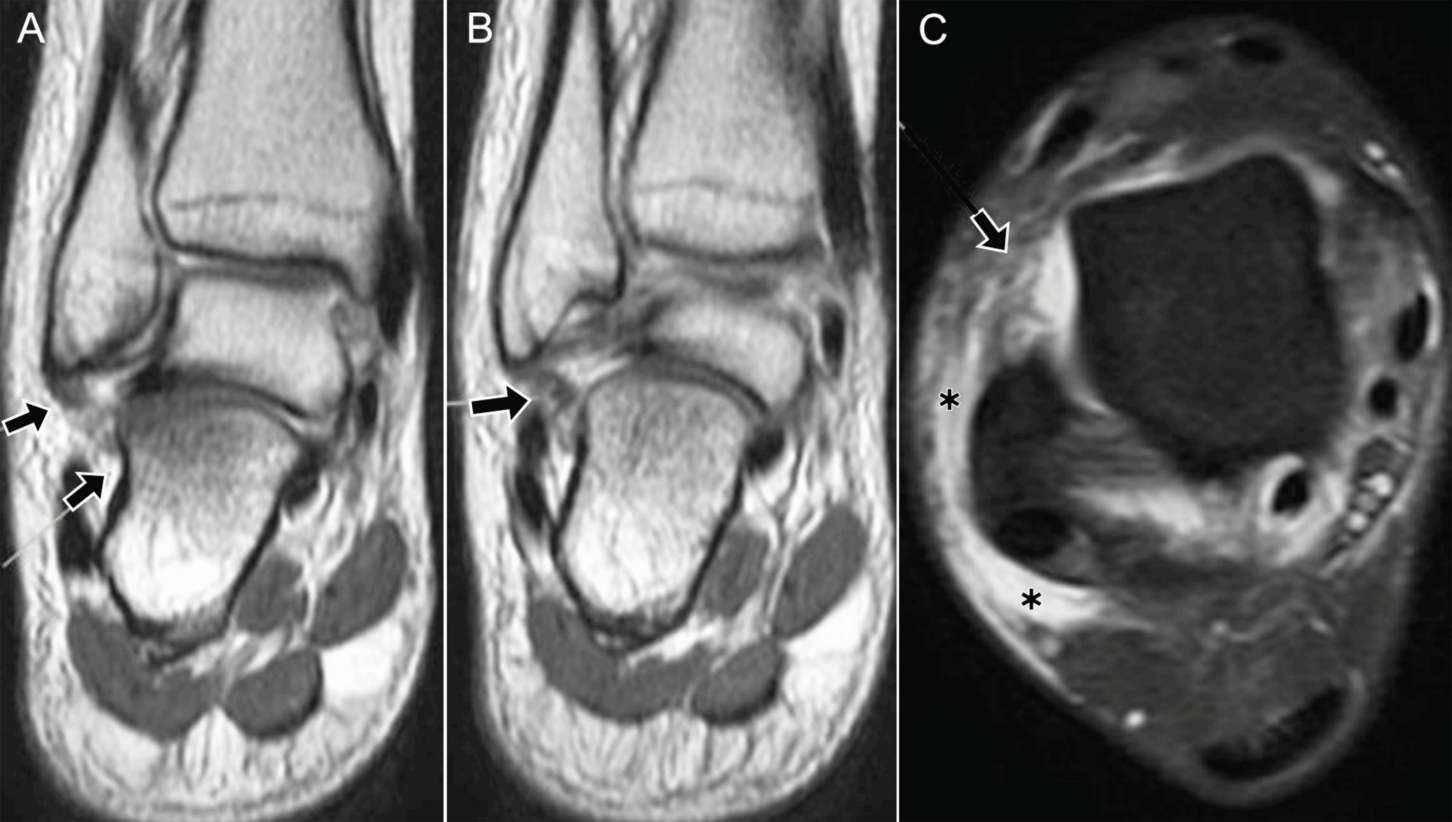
Calcaneofibular and ATFL tears after trauma. A 22-year-old female: (A, B) coronal DP MRI (double-echo proton) reveals full-thickness CFL tears (arrows); (C) axial DPFS MRI demonstrates a complete ATFL tear (arrow) and lateral soft-tissue injury (*). CFL: calcaneofibular ligament; DPFS: dual proton fat-saturated sequence; ATFL: anterior talofibular ligament Images belong to the authors; patient consent was obtained.
